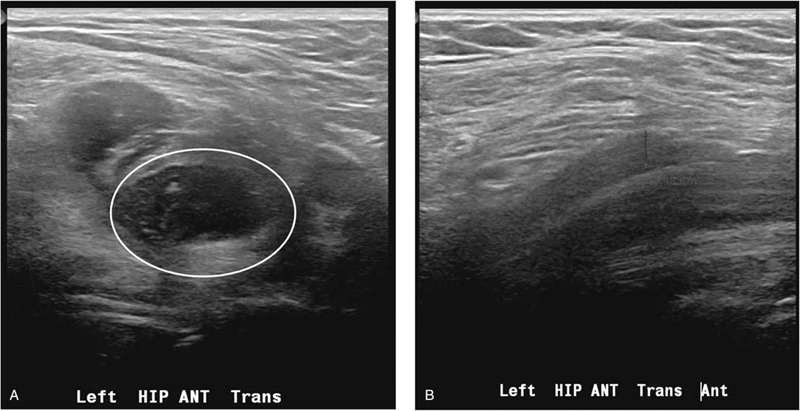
Ultrasonography-guided aspiration of the left iliopsoas hematoma. Approximately 10 cc of dark bloody fluid was aspirated (red circle, revealed hematoma in the iliopsoas muscle). (A) The white circle indicates the hematoma at the iliopsoas muscle on ultrasonography. (B) After removal of the hematoma, the thickness of the left iliopsoas hematoma decreased 0.3 cm.
